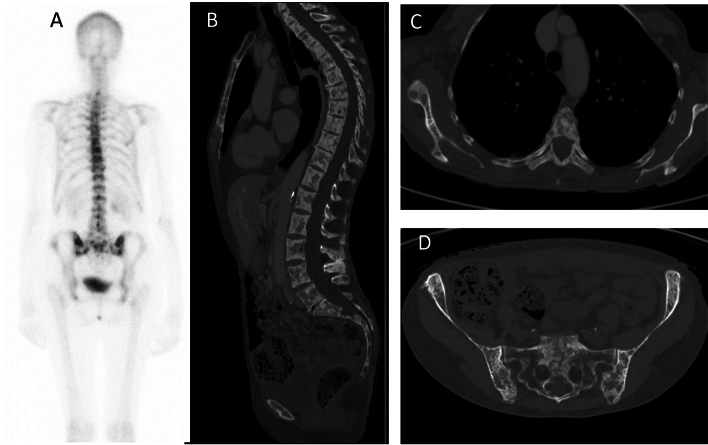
Imaging studies. A 99mTc-DPD scintigraphy showed multiple foci of increased uptake: B–D CT scan
